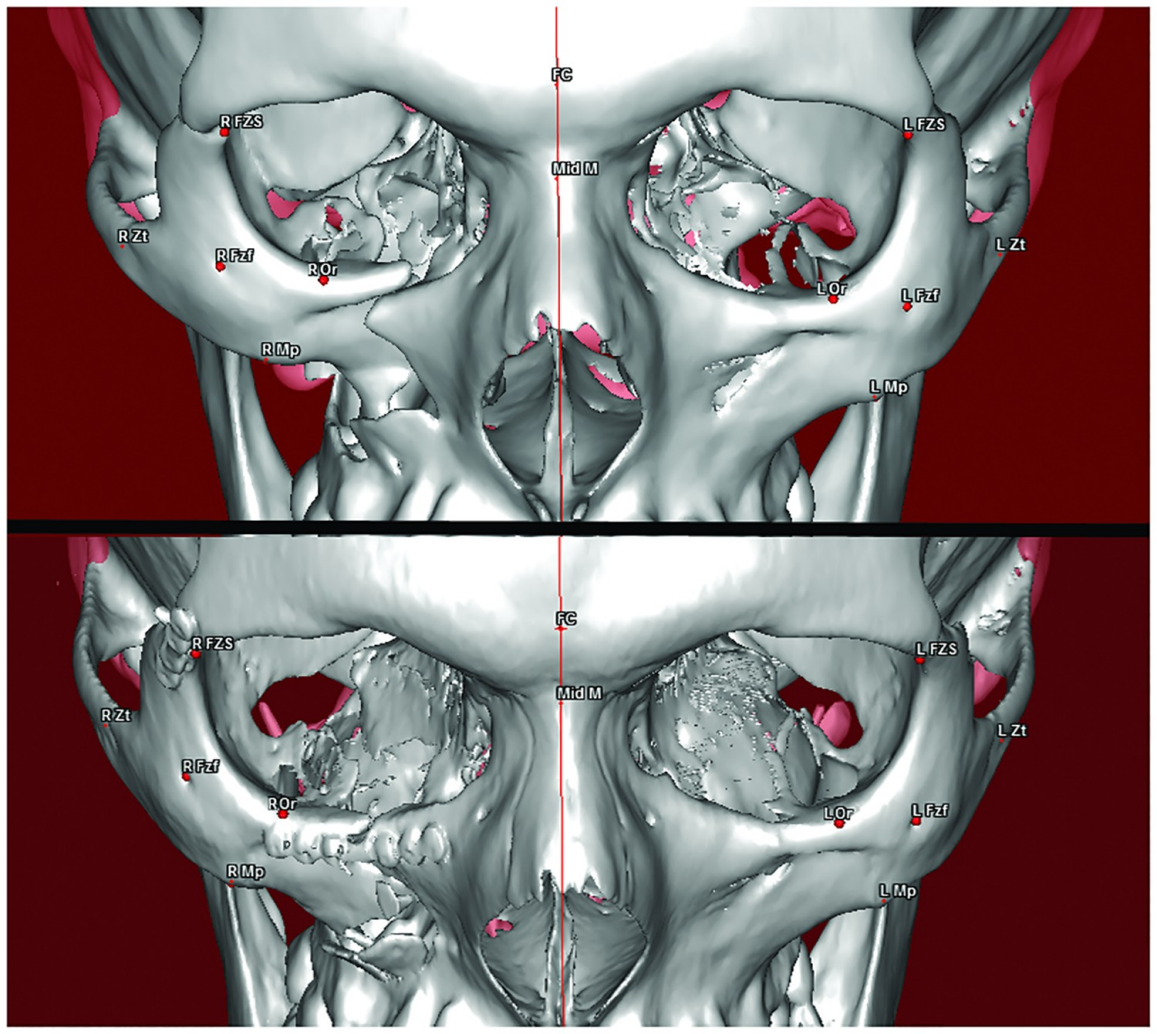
Three-dimensional reconstruction of the CT scan in a patient with a right tetrapodal ZMC fracture treated by the ORIF technique (lower orbital rim and fronto-zygomatic miniplates), preoperatively (top), and postoperatively (bottom) showing good radiographic outcomes in terms of the bone reduction.
